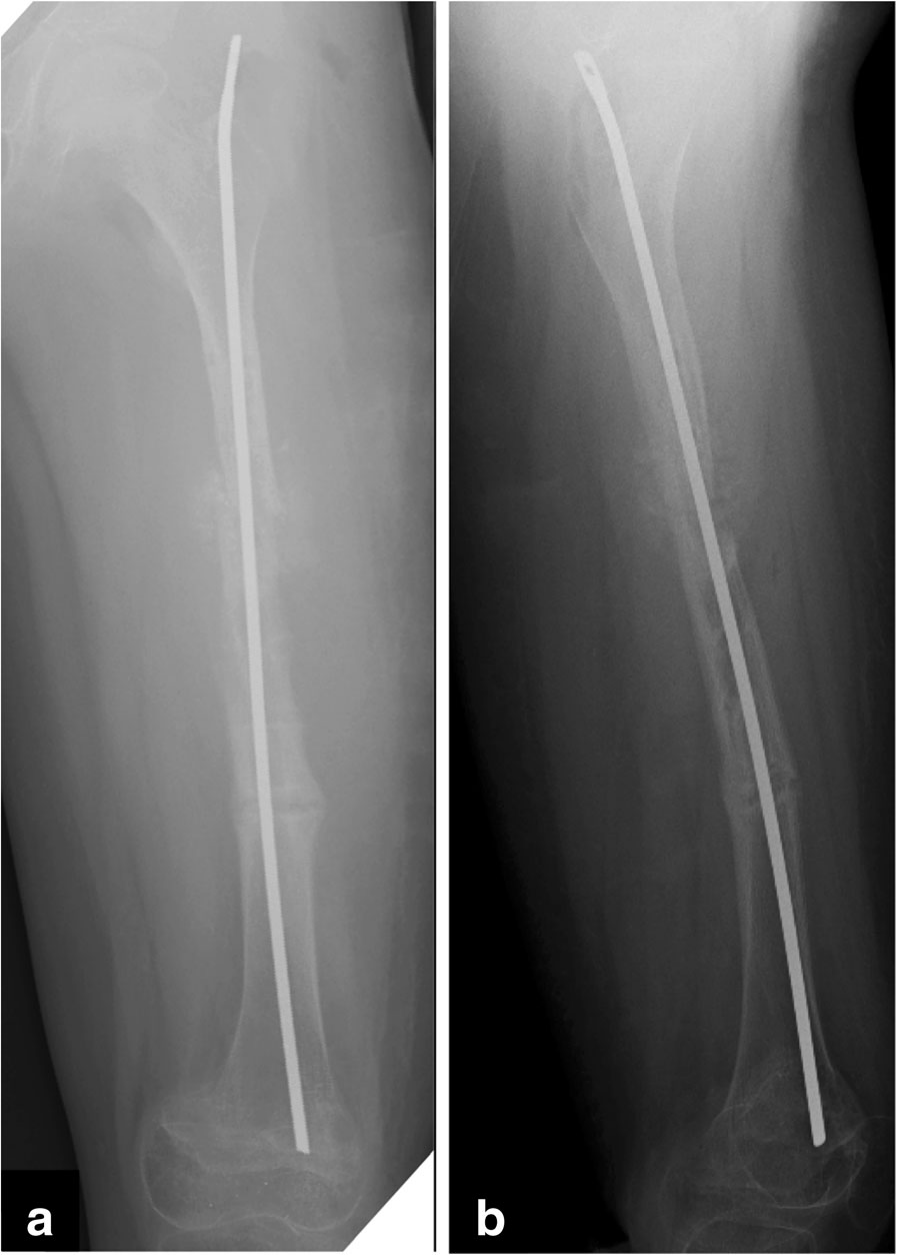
Radiographs of the left femur after the revision surgery with the Ender nail. a the anteroposterior view, b the lateral view
